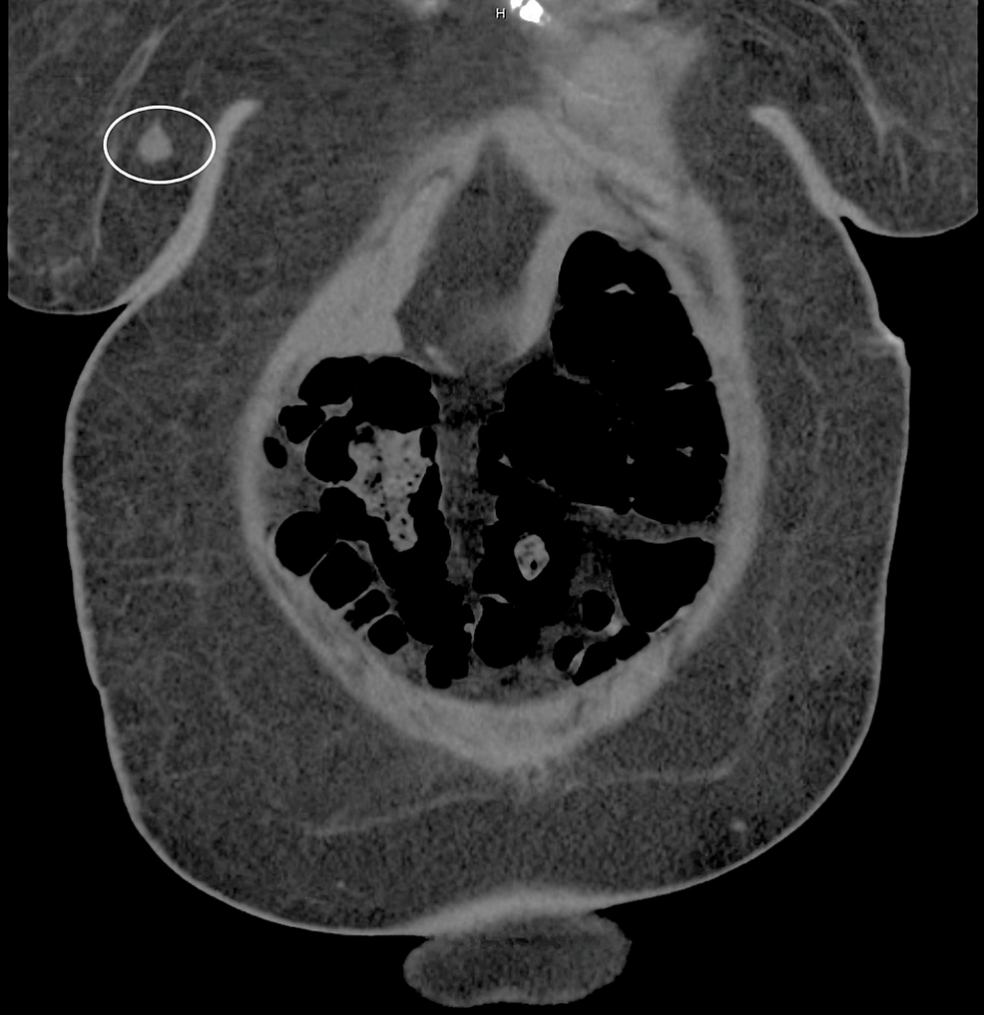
CT abdomen and pelvis. CT scan of the abdomen and pelvis demonstrating evidence of a right breast mass (circled). CT: computed tomography
